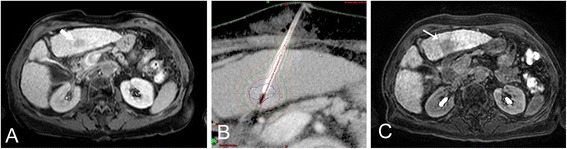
Magnetic resonance imaging scan of a 69-year-old patient with liver cirrhosis and hepatocellular carcinoma in liver segment III (a, arrow). The tumor was treated by CT-guided high-dose rate brachytherapy with a single catheter and a tumor-enclosing target dose of 20 Gy (b). Six weeks later, complete tumor ablation is indicated by a surrounding lack of uptake of hepatocyte-specific contrast medium (Primovist™, Bayer Healthcare, Leverkusen, Germany) (c, arrow)
